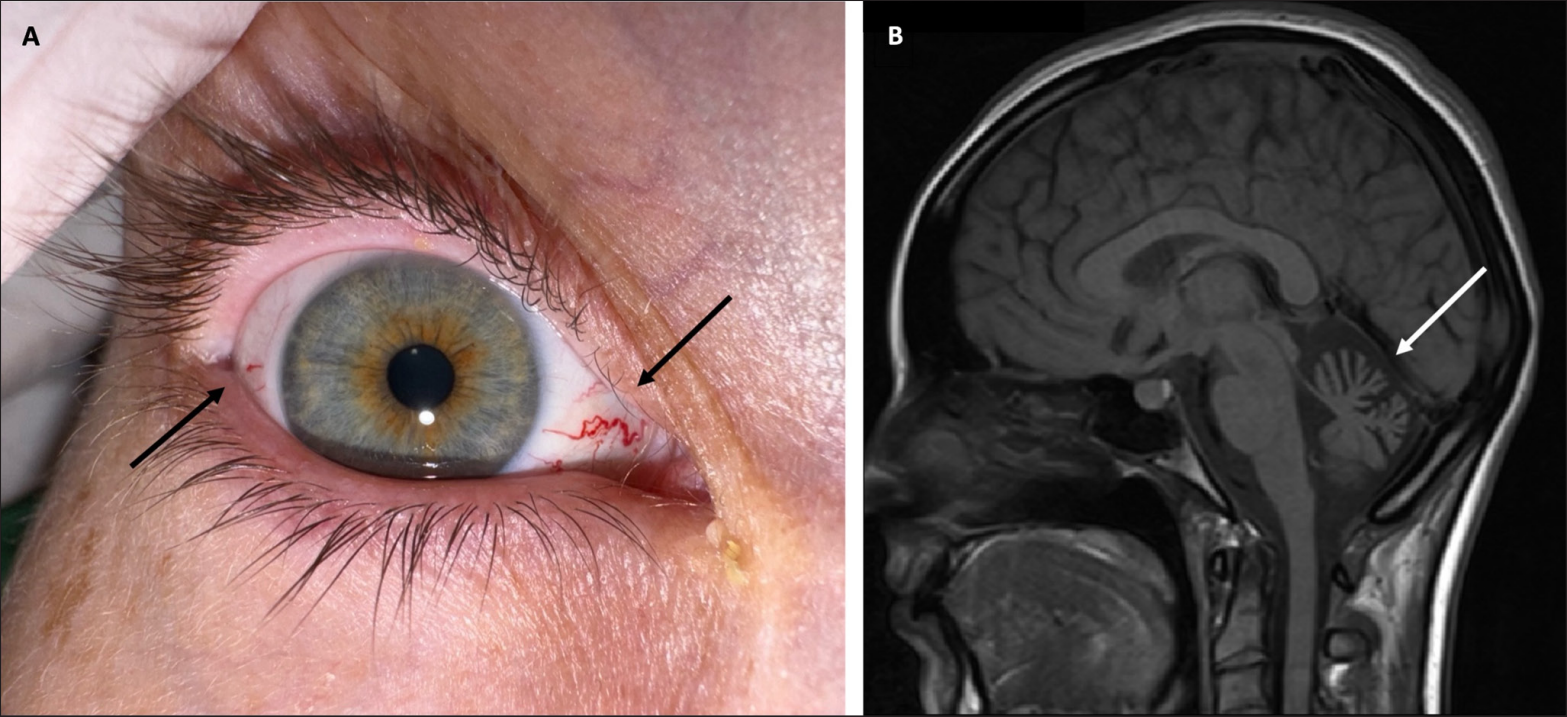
Ocular telangiectasias and cerebellar atrophy. Ocular telangiectasias (black arrows) in the patient’s right eye (A) and atrophy of the cerebellar vermis (white arrow) in T1-weighted sagittal cerebral magnetic resonance imaging (B), representing the eponymous features of ataxia-telangiectasia - small, dilated blood vessels in the sclera and ataxia due to progressive cerebellar degeneration.
